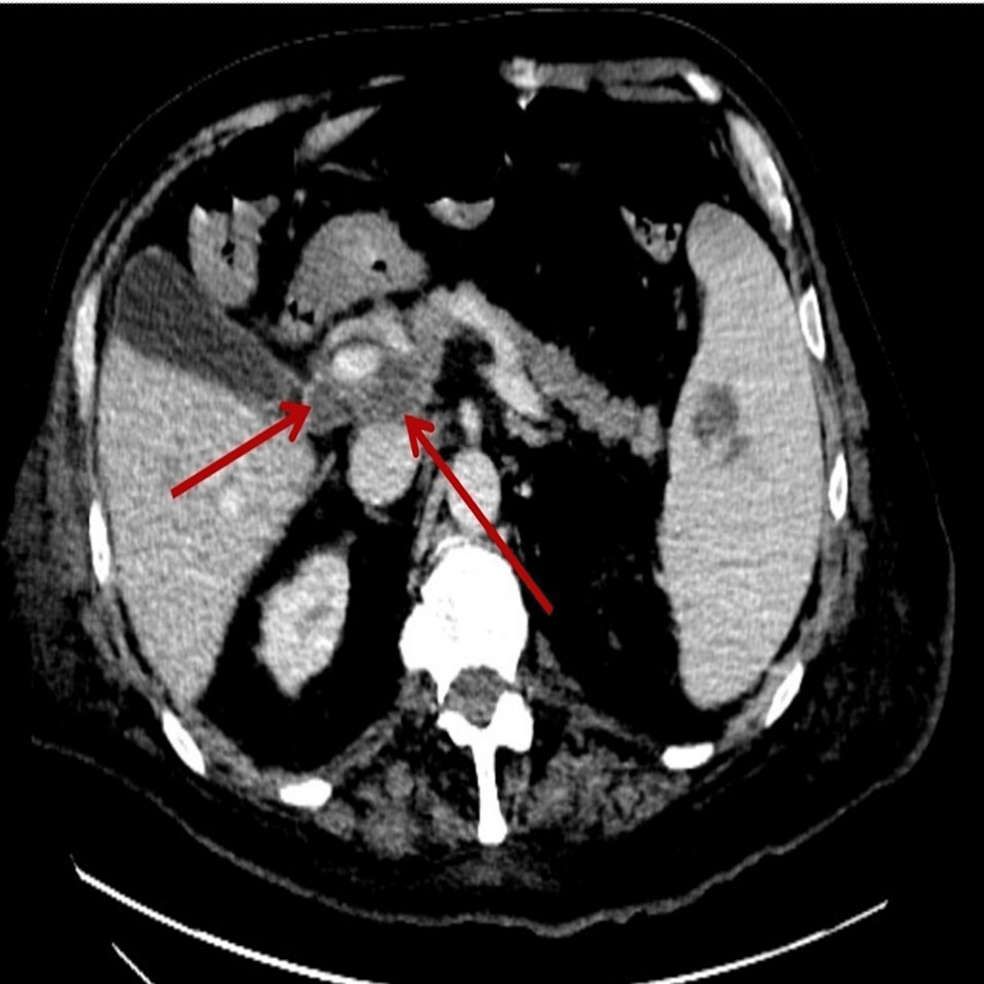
CT abdomen axial view revealing a large portocaval lymph node (arrows) and low attenuation suggesting a large necrotic node with splenic lesion
